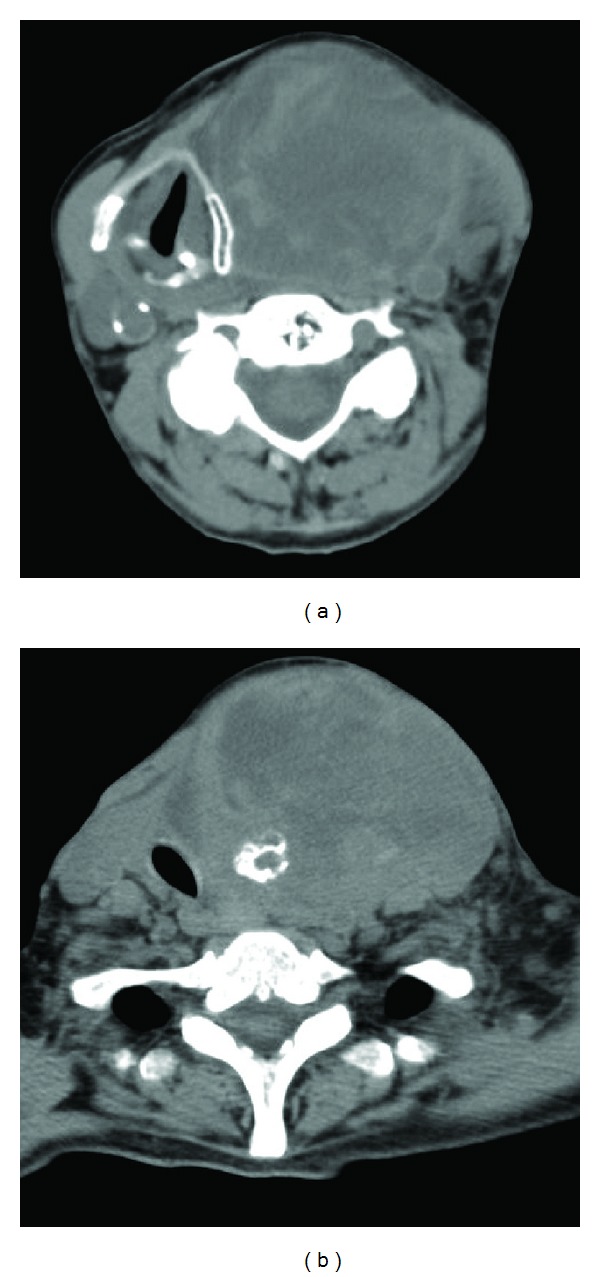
(a) Axial CT image showing the displacement of the larynx to the right by a heterogenous swelling on the left; (b) displacement of the trachea to the right by the lesion with internal calcification.
